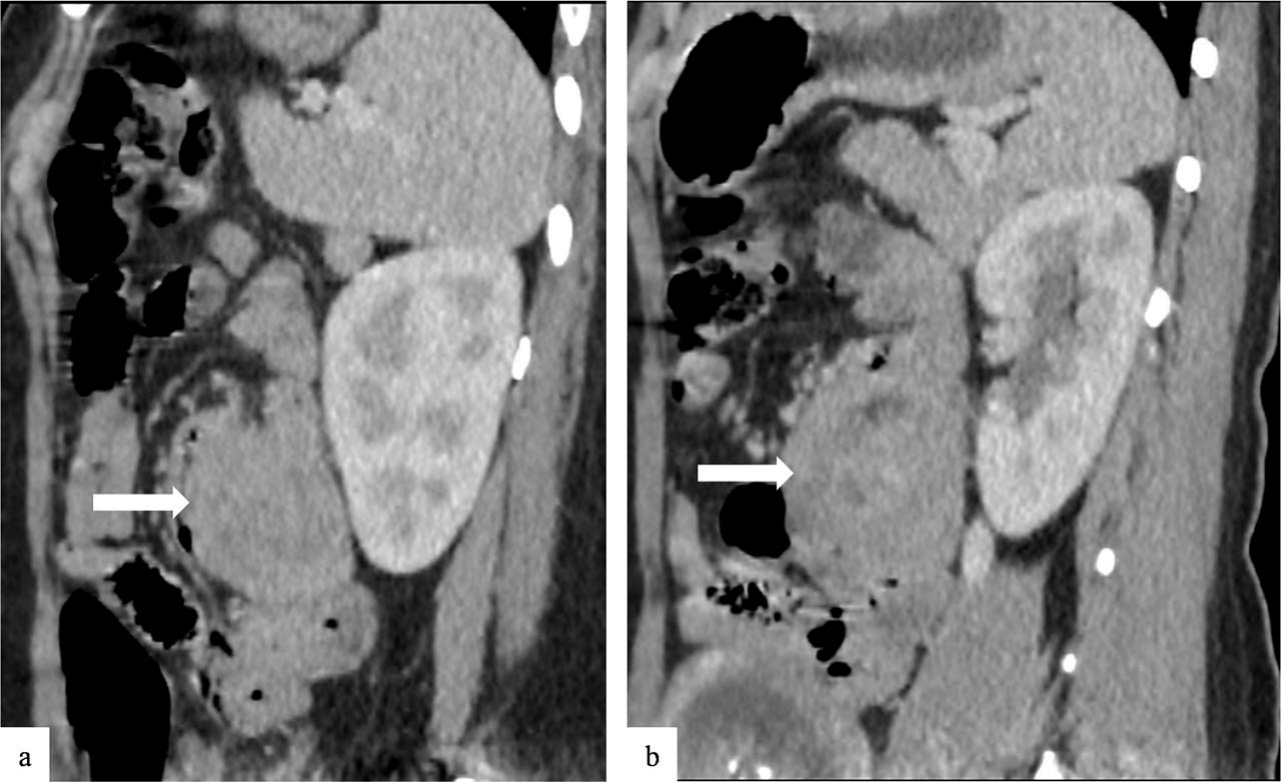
Sagittal contrast-enhanced CT images (a, b) showing the duodenojejunal intussusception with a pseudokidney appearance (arrows).
